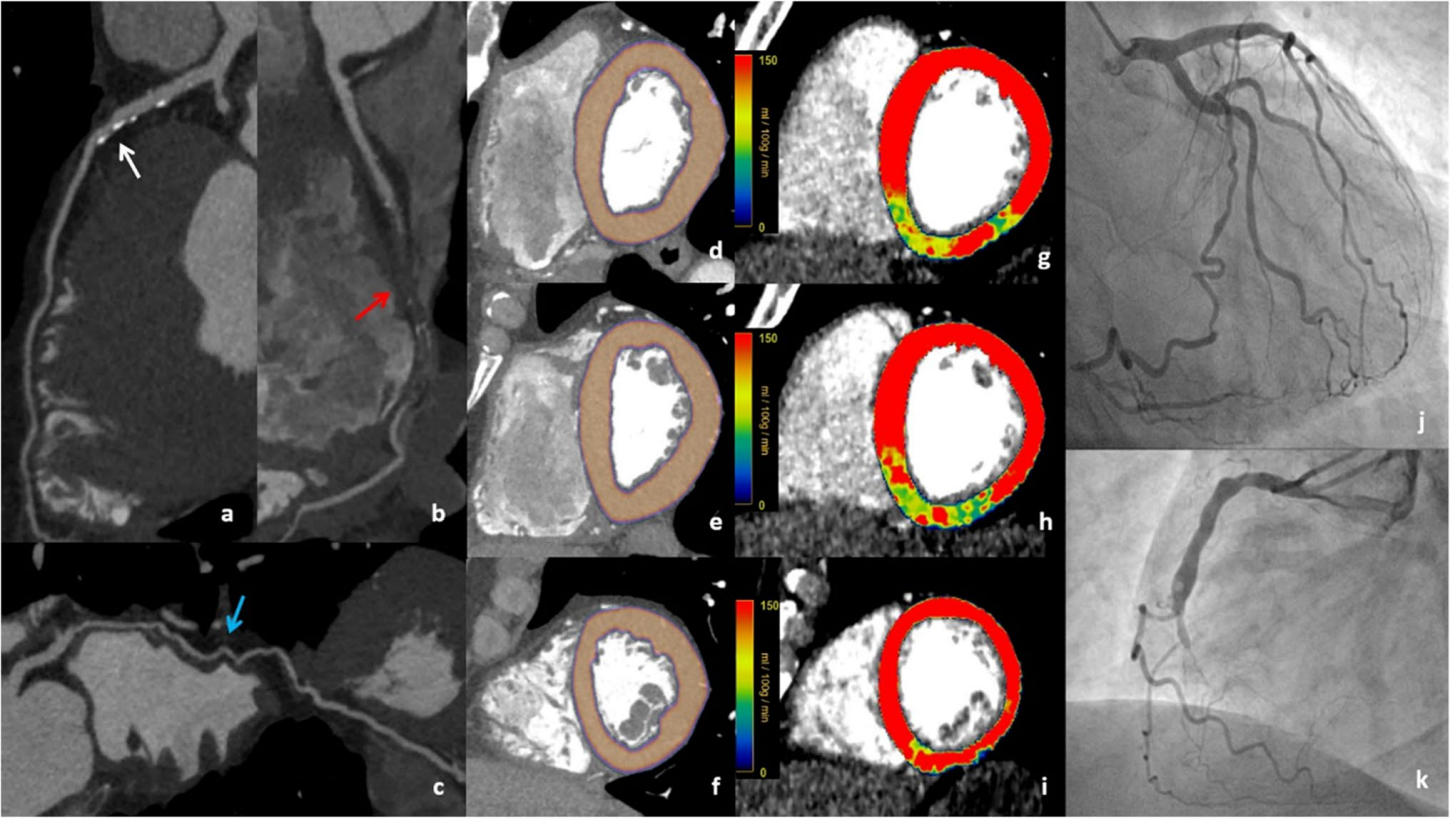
A 60-year-old man with recent onset of effort dyspnea. Coronary CTA was performed, with the evidence of moderate mid-LAD stenosis (a, white arrow), chronic total occlusion of mid-RCA (b, red arrow), and the presence of collateral supply for RCA from LCX (c, blue arrow). Rest myocardial perfusion assessment revealed a fully viable myocardium (d, e, f). After adenosine administration, a stress CTP scan performed with dynamic acquisition showed inducible ischemia at the basal to the mid inferior septum, basal to the mid-inferior wall, and basal inferolateral wall (g, h, i). The absence of relevant coronary stenosis at LAD, presence of collateral supply from LCX, and chronic total occlusion of mid-RCA were confirmed at invasive assessment (j, k).CTA: computed tomography angiography; LAD: left anterior descending; RCA: right coronary artery; LCX: left circumflex, CTP: computed tomography perfusion
